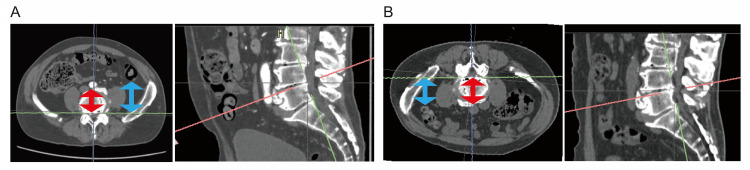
CT images of the lumbar spine in supine and prone positions (A) Left: CT image perpendicular to the L4/5 intervertebral disc space in supine position. Right: Sagittal reconstruction image. Blue arrows indicate the distance of the bowel at the left side to the posterior vertebral line. Red arrows indicate the distance of the abdominal aorta to the posterior vertebral line. (B) Left: CT image perpendicular to the L4/5 intervertebral disc space in prone position. Right: Sagittal reconstruction image. Blue arrows indicate the distance of the bowel at the left side to the posterior vertebral line. Red arrows indicate the distance of the abdominal aorta to the posterior vertebral line. CT: computed tomography
